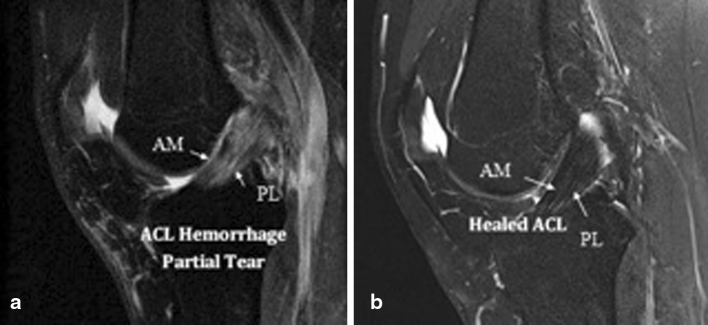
a MRI showing AM bundle isolated tear. b Six months later, MRI showing complete healing
